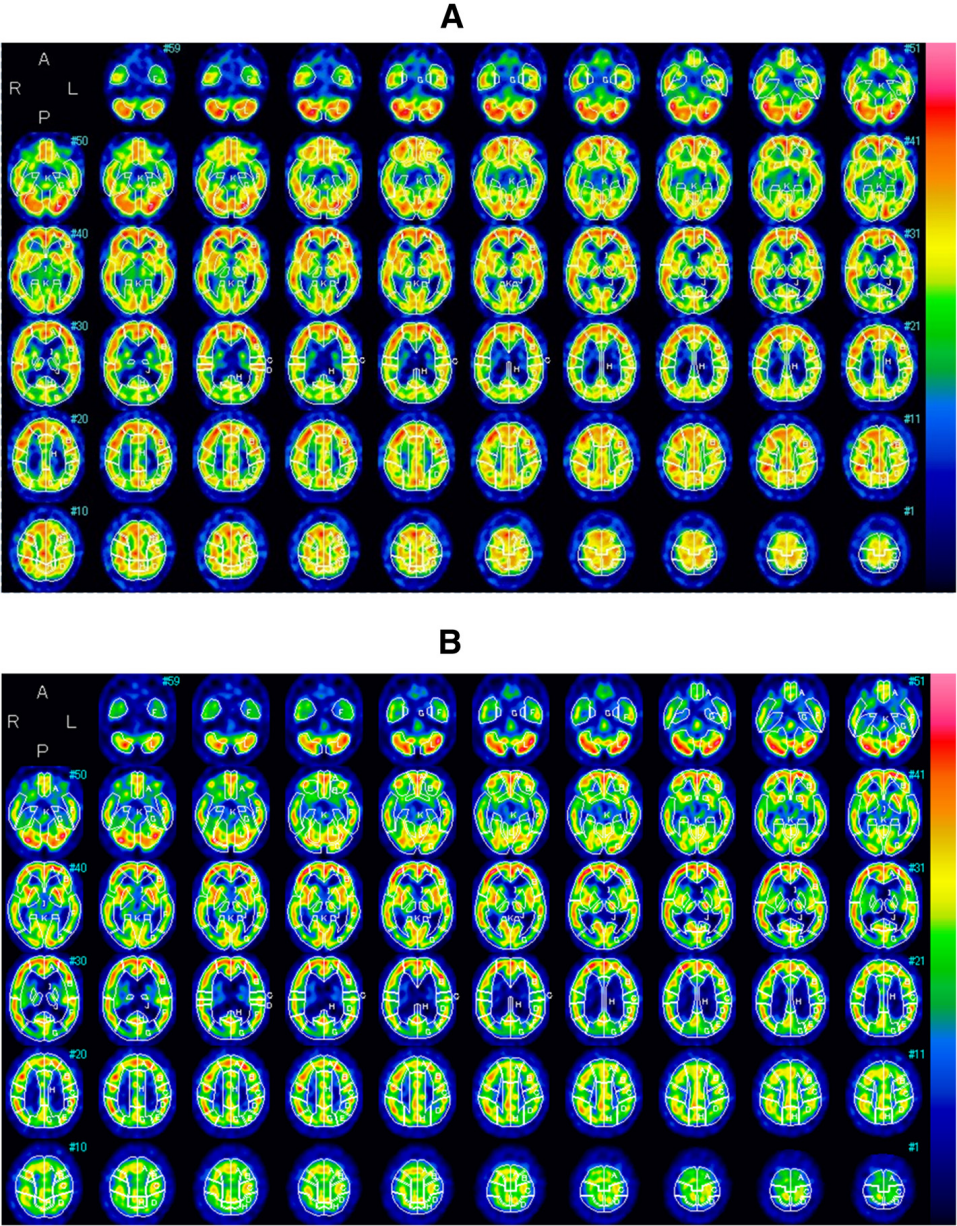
eZIS from ictal and interictal Tc-99 m ECD-SPECT (A), (B).
